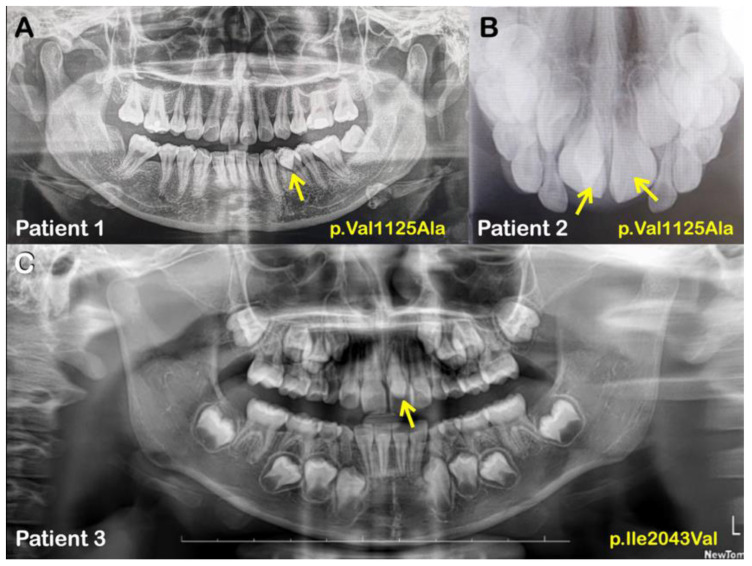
(A) Patient 1. Panoramic radiograph showing a supernumerary left mandibular premolar (arrow). (B) Patient 2. Occlusal radiograph showing double mesiodentes (arrows). (C) Patient 3. Panoramic radiograph showing a mesiodens (arrow).
